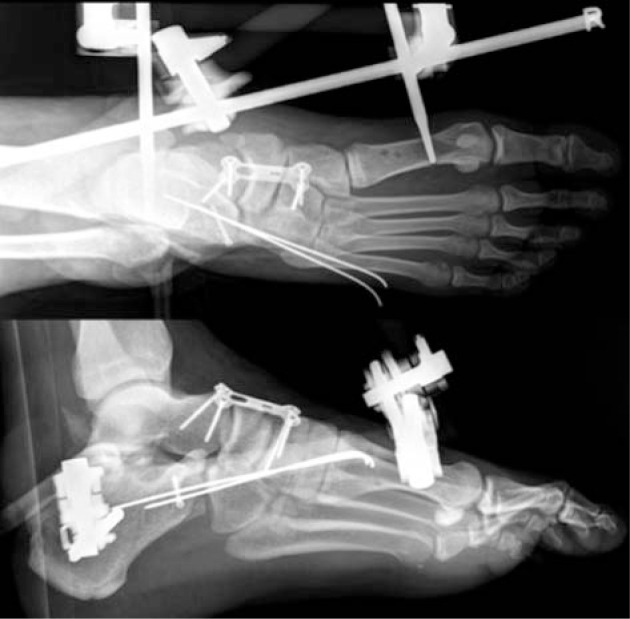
Immediate post-operative radiographs showing good reduction of the calcaneocuboid and naviculocuneiform joints. The external fixator was left in-situ for additional stability.
